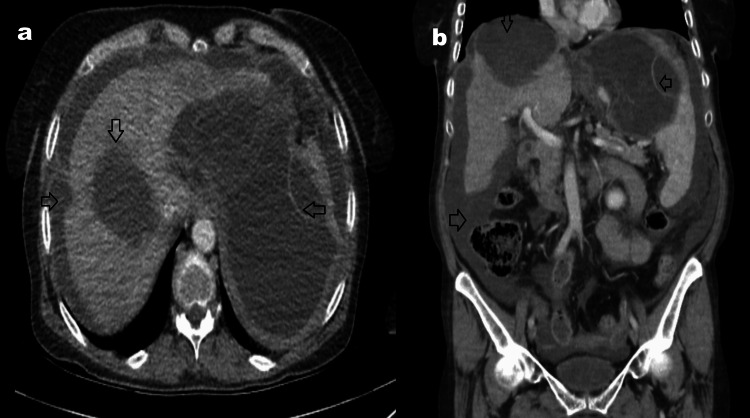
CT images taken in the emergency room. CT images obtained upon the patient's admission to the emergency department showed that the cyst wall in the right hepatic lobe (a, b) remained relatively intact, while the cyst in the left lobe had decreased in size. There was also widespread free fluid in the abdominal cavity. It was noted that the free fluid had the same density (b) as the cyst contents. Cyst in the right hepatic lobe (arrow ∨); volume loss and perforation findings in the cyst on the left (arrow <); free fluid in the abdomen (arrow >).
